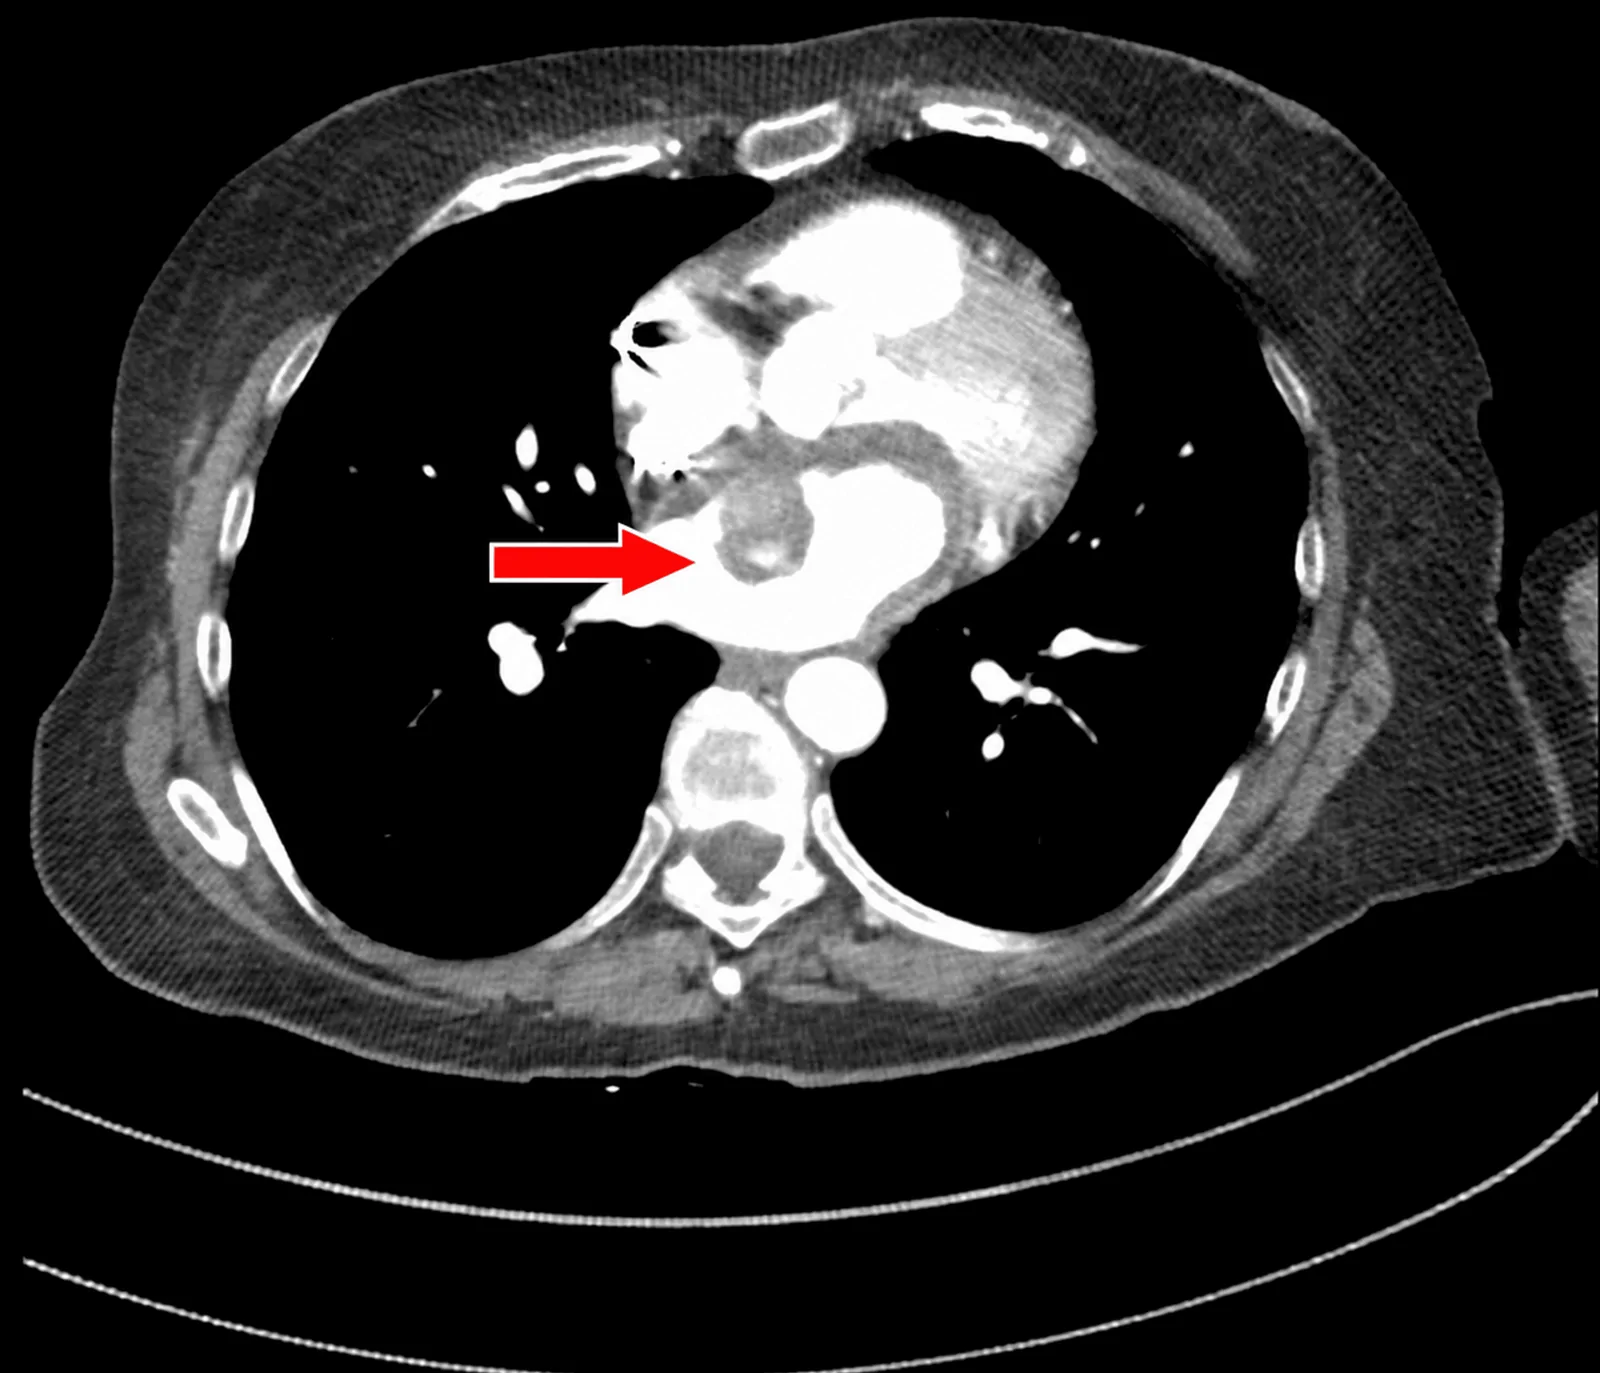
CT angiography of the chest demonstrating the mass. Axial image demonstrates a large, heterogeneous hypoattenuating mass within the contrast-opacified left atrium (arrow), occupying a substantial portion of the atrial cavity.
